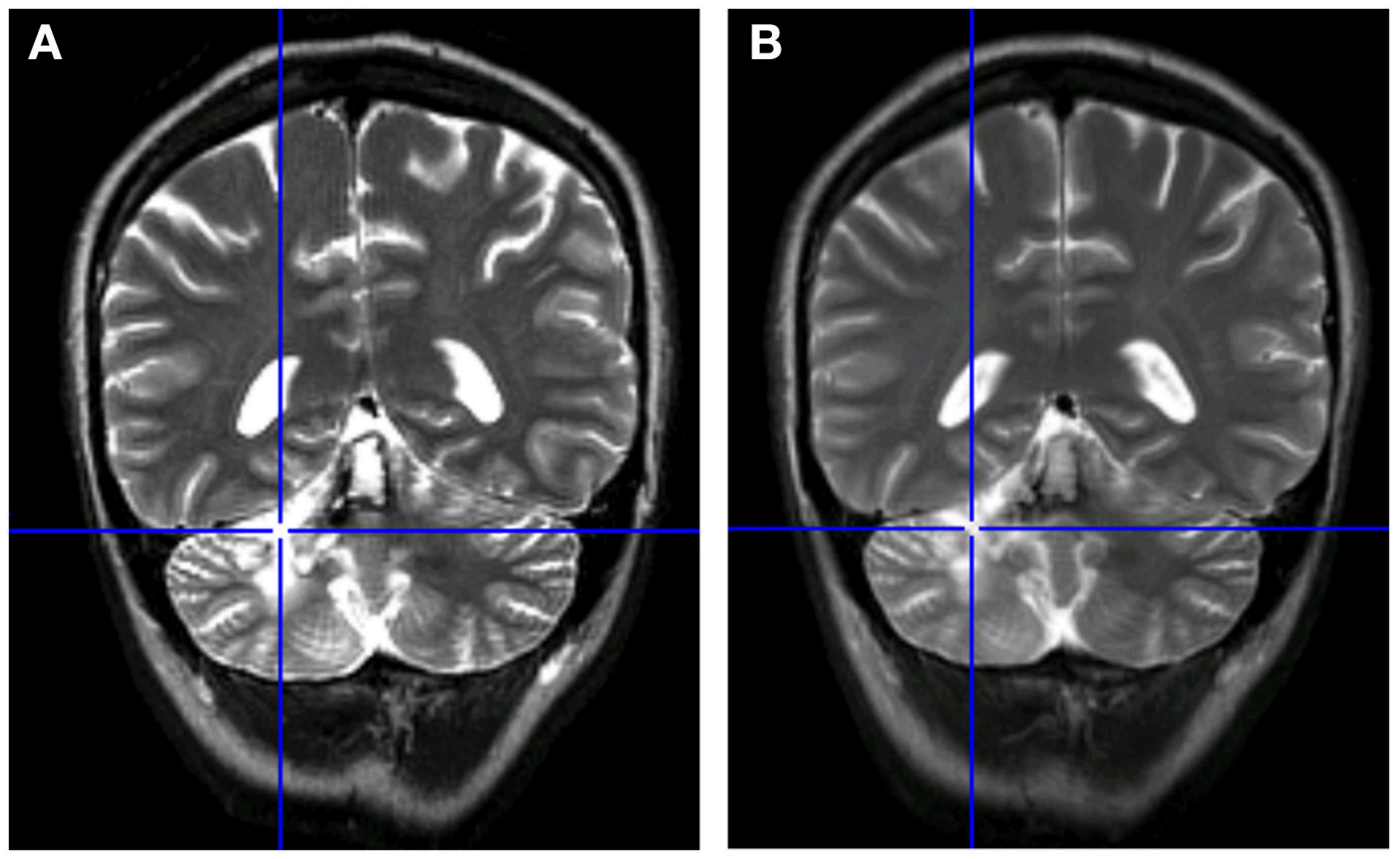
Comparison between the patient's MRI scans. (A) MRI in May 2015 (10 months after acute event of July 2014). (B) MRI at follow-up in January 2016 (8 months after the initial scan).
